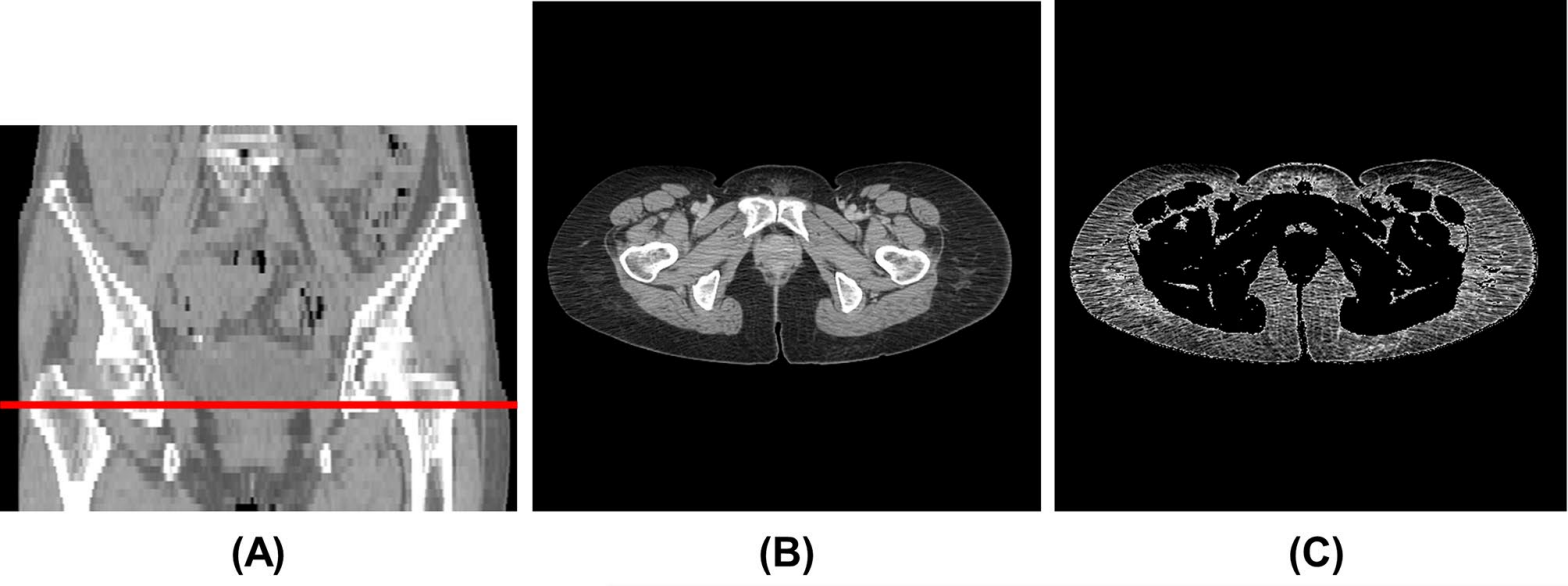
Example of CT image at the greater trochanter level. (A) Coronal section (red line: greater trochanter level), (B) original image (window level, 50 HU; window width, 400 HU), (C) fat-enhanced image.
